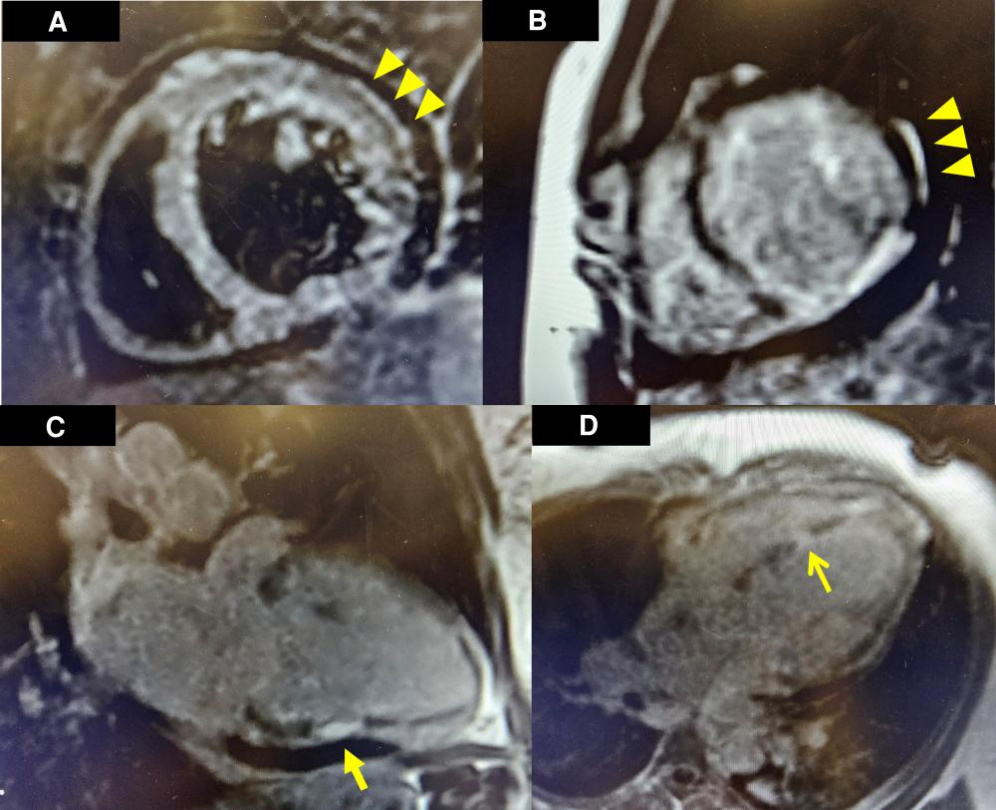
Cardiac magnetic resonance imaging findings. Subepicardial oedema can be visualized in the basal anterolateral segment of the left ventricle in T2 short-tau inversion recovery imaging (*A*). On post-contrast T1-prepared images, late gadolinium enhancement was observed in a corresponding and similar distribution of the basal anterolateral segment (*B*). Additional full thickness late gadolinium enhancement was noted in the midsegment of the inferior wall in a two-chamber view (*C*). Full thickness late gadolinium enhancement was noted in the mid inferoseptal segment of the left ventricle in a four-chamber view (*D*).
